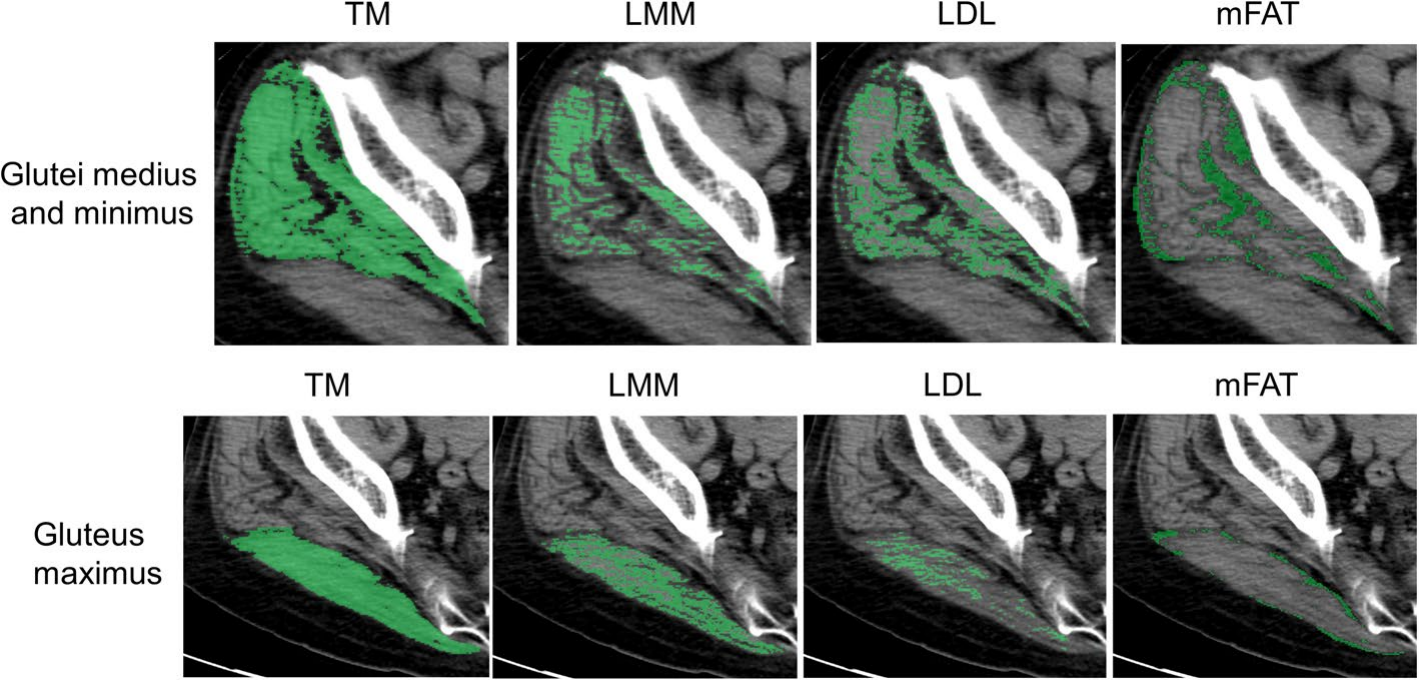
Measurement of muscle composition on an axial image of computed tomography. Total muscle cross-sectional area (TM) of the glutei medius and minimus or the upper portion of the gluteus maximus is segmented using the threshold of − 29 to 150 Hounsfield units (HU). Lean muscle mass (LMM), low-density lean tissue (LDL), and intramuscular fat (mFAT) are colored as the pixels with the density of 30 to 80 HU, 0 to 29 HU, and − 190 to − 30 HU, respectively, within each segmented TM
